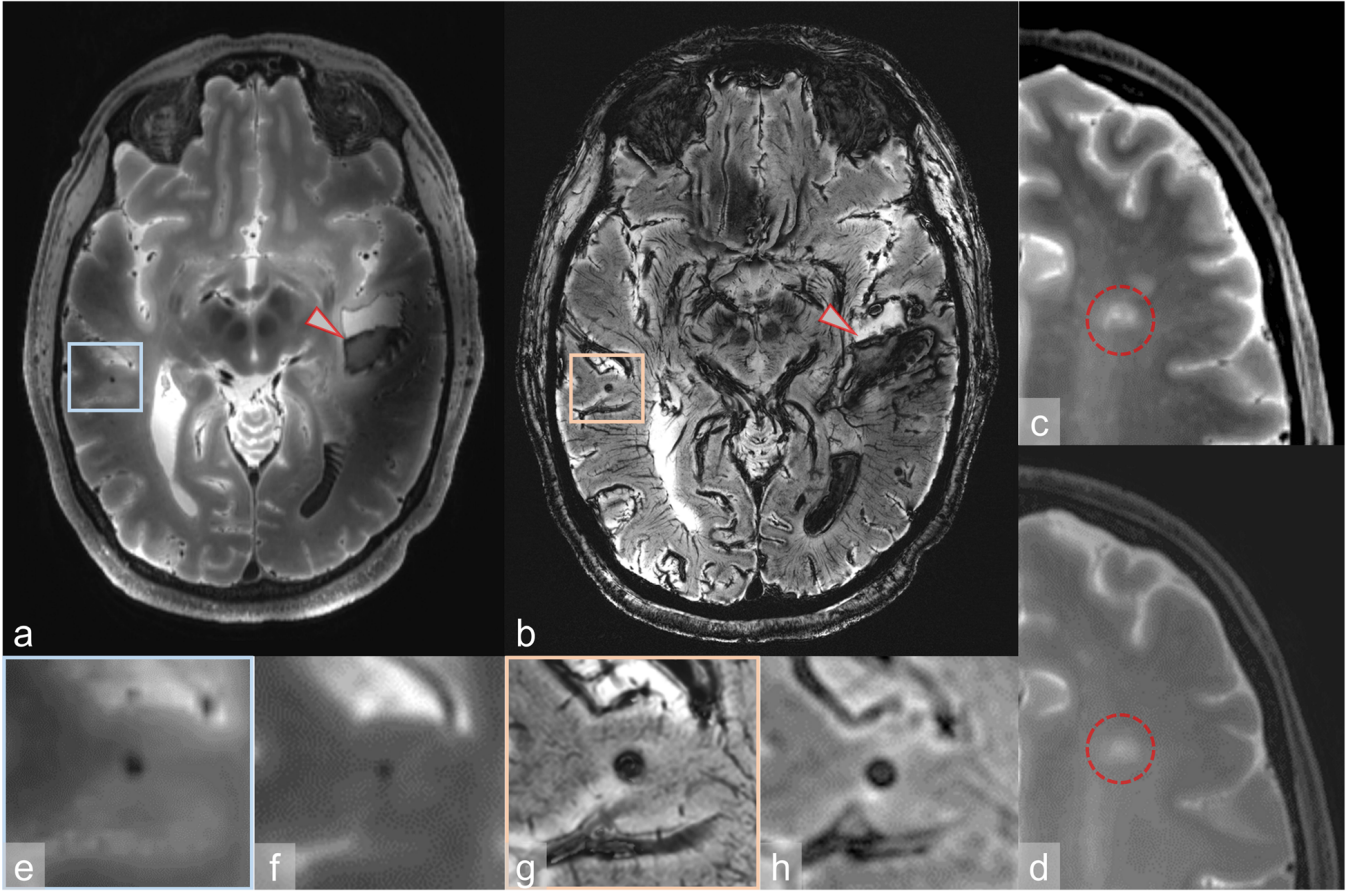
a-h Case 2. An intraparenchymal haemorrhage was delineated on 7-T MRI (arrowhead in a: T2-weighted sequence (sampling perfection with application-optimised contrasts by using different flip angle evolutions, SPACE) and in b: susceptibility-weighted imaging). The haemorrhage presented with postmortem sedimentation. The SPACE image is limited by signal inhomogeneity visible on the anatomically left side adjacent to the haemorrhage. In addition, MRI detected subtle lesions, better delineated at 7 T than at 3 T: microinfarcts (c: 7-T T2-weighted SPACE sequence; d: 3-T T2-weighted turbo field echo sequence), and microhaemorrhages (e and g 7-T T2-weighted SPACE sequence and 7-T susceptibility-weighted imaging; f and h, 3-T T2-weighted turbo field echo sequence and venous blood oxygen level-dependent imaging)
